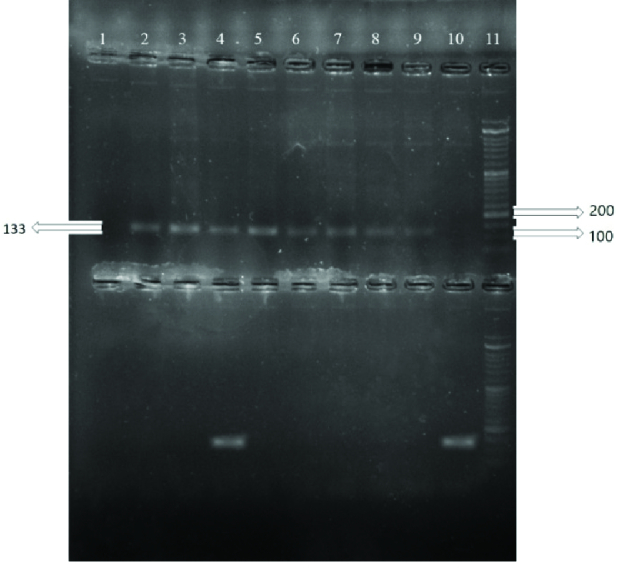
ARMS-PCR products of rs28371725. The top bands show wild alleles and the down bands show mutant alleles for each sample: Number 1 is negative control; 2, 3, 5, 6, 7, 8 and 9 are wild homozygotes (CC), 4 is heterozygote (CT), 10 is mutant homozygote (TT), and 17 is 50 bp DNA ladder.
